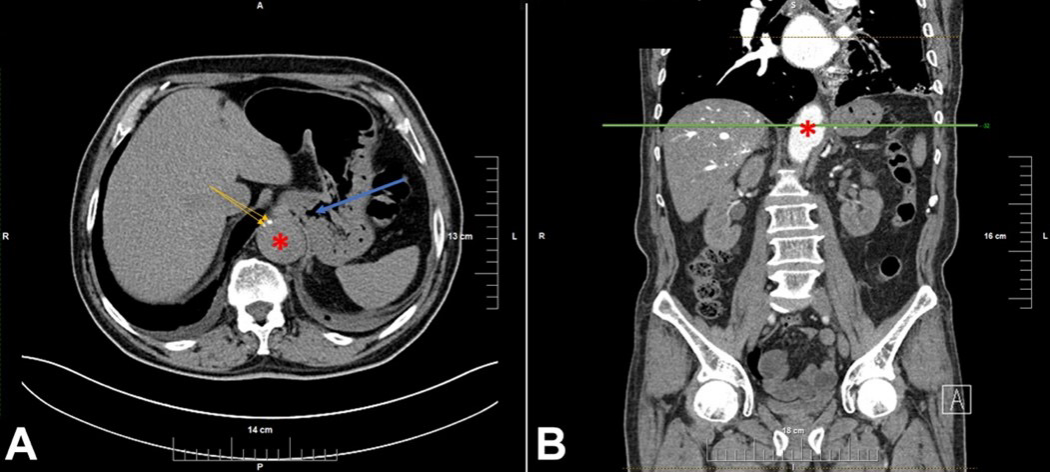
Thoracoabdominal CT scan: A – red asterisk indicates the abdominal aortic aneurysm, yellow arrows indicate the calcium aggregated in the atherosclerotic plaque, blue arrow points toward the duodenal lumen and the duodenal wall adjacent to the aneurysm; B – red asterisk indicates the lumen of the aneurysm, the green line indicates the level of section A of the figure. CT: computer tomography.
